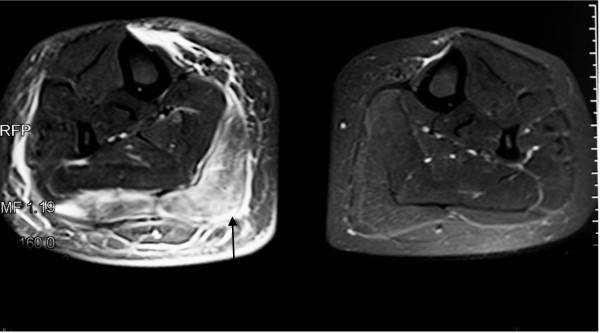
Magnetic resonance imaging images of both legs. Increased signal intensity in the T2-weighted image of the right gastrocnemius muscle and subcutaneous tissue (arrow) was caused by severe inflammatory changes, whereas the left leg is normal.
